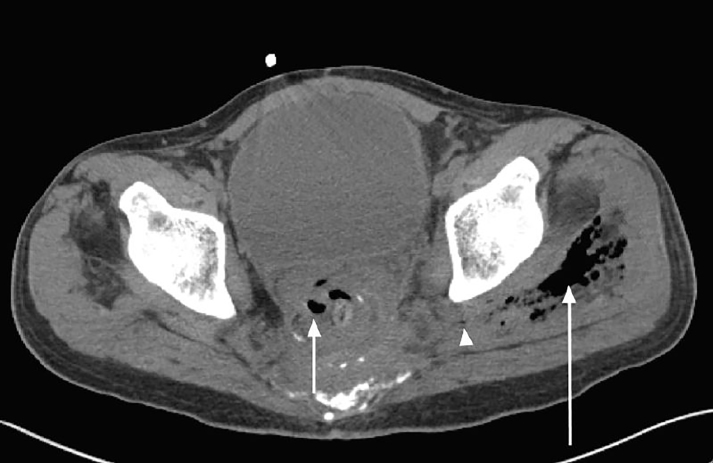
Axial computed tomography of the abdomen and pelvis demonstrating air and fluid in the pelvis (short arrow), exiting the sciatic notch (arrowhead) into the left buttock, with a large air and fluid collection centered in the left middle gluteal muscle (long arrow).
